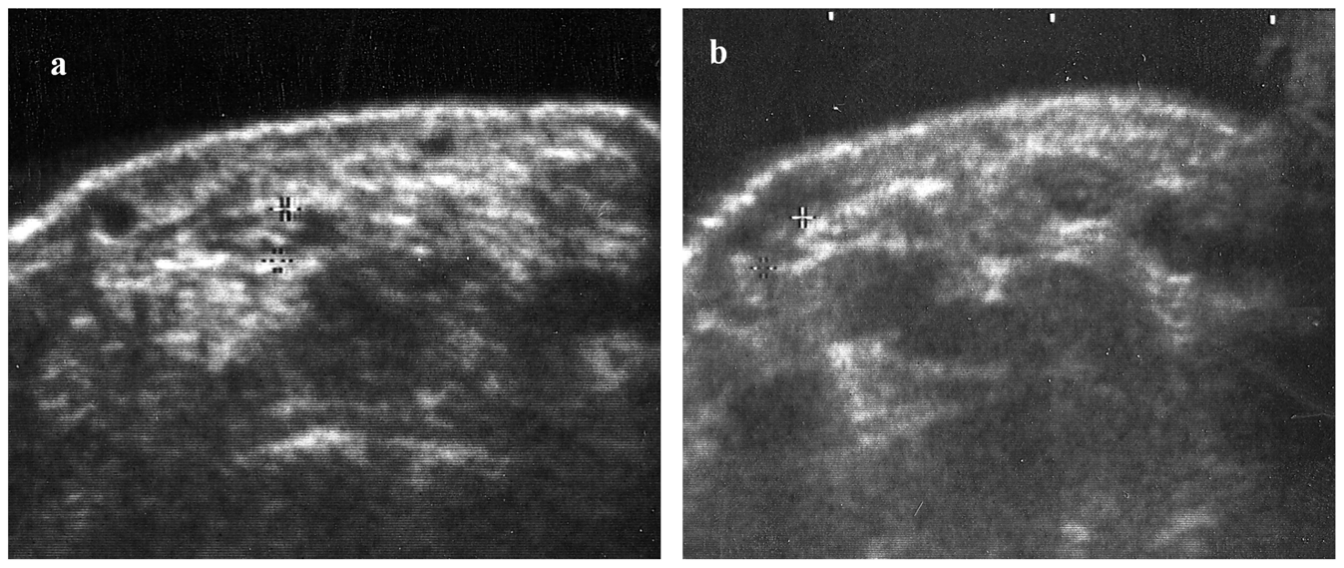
Ultrasonographic images. (a) View of the carpal tunnel with fingers at rest position. (b) View of the carpal tunnel with fingers at flexion position. Note median nerve movement in ulnar direction (from the left mark to the right) and movement of the FDS and FDP of the index and long fingers in radial direction.
